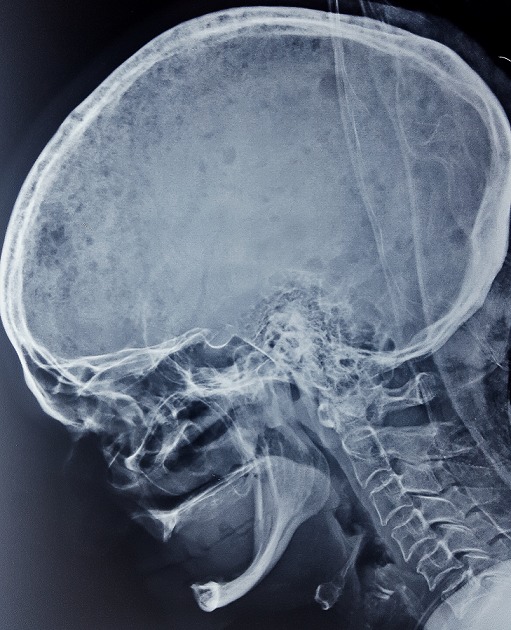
Radiographie du crâne: lésions lacunaires en « emporte-pièces » caractéristiques du myélome multiple
